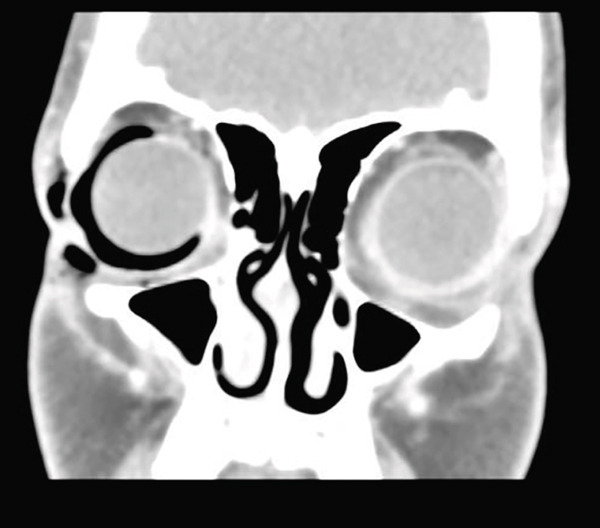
Computed tomography (CT) of the orbit demonstrated a localized collection of air closely following the contour of the globe, consistent with the sub‐Tenon space. There was no evidence of orbital fracture or sinus communication. Periorbital subcutaneous emphysema was also present.
